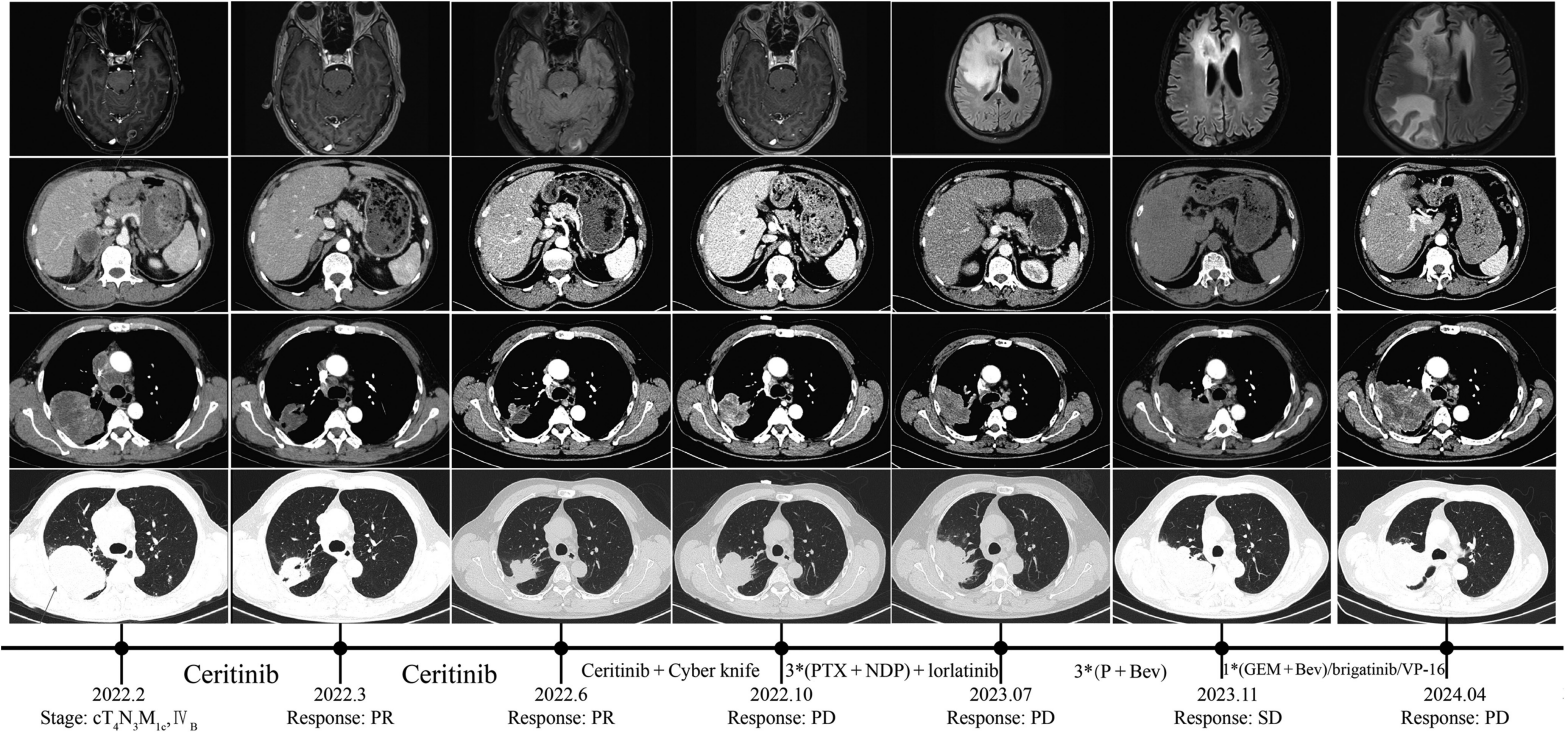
CT and MRI scans of Case 3 before and after treatment. Ceritinib: 450 mg orally per day; Ceritinib + Cyber knife: ceritinib 450 mg orally per day and brain lesion radiotherapy (dose: 33Gy/3F). Bev, bevacizumab; GEM, gemcitabine; NDP, nedaplatin; P, pemetrexed; PD, progressive disease; PR, partial remission; PTX, paclitaxel; SD, stable disease; VP16, etoposide. The red arrows point to primary lung cancer, metastatic lymph nodes, and metastatic lesions.
